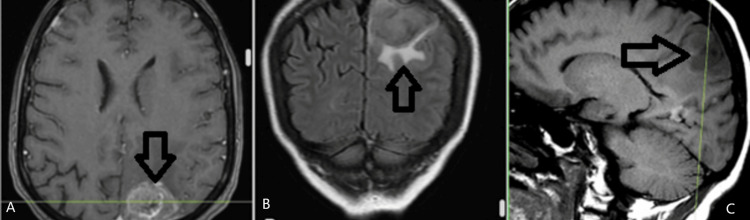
Left parietal lobe lesion imaged through MRI A: axial plane; B: coronal plane; C: sagittal plane
